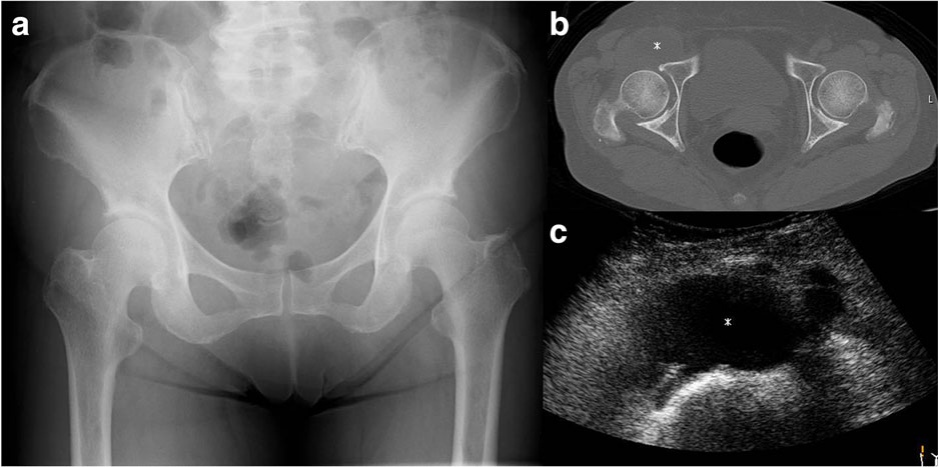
a An initial anteroposterior radiograph showed no significant findings. Computed tomography (b) and ultrasonography (c) showed a cystic mass (asterisk) arising from the right hip joint and compressing the femoral vein
